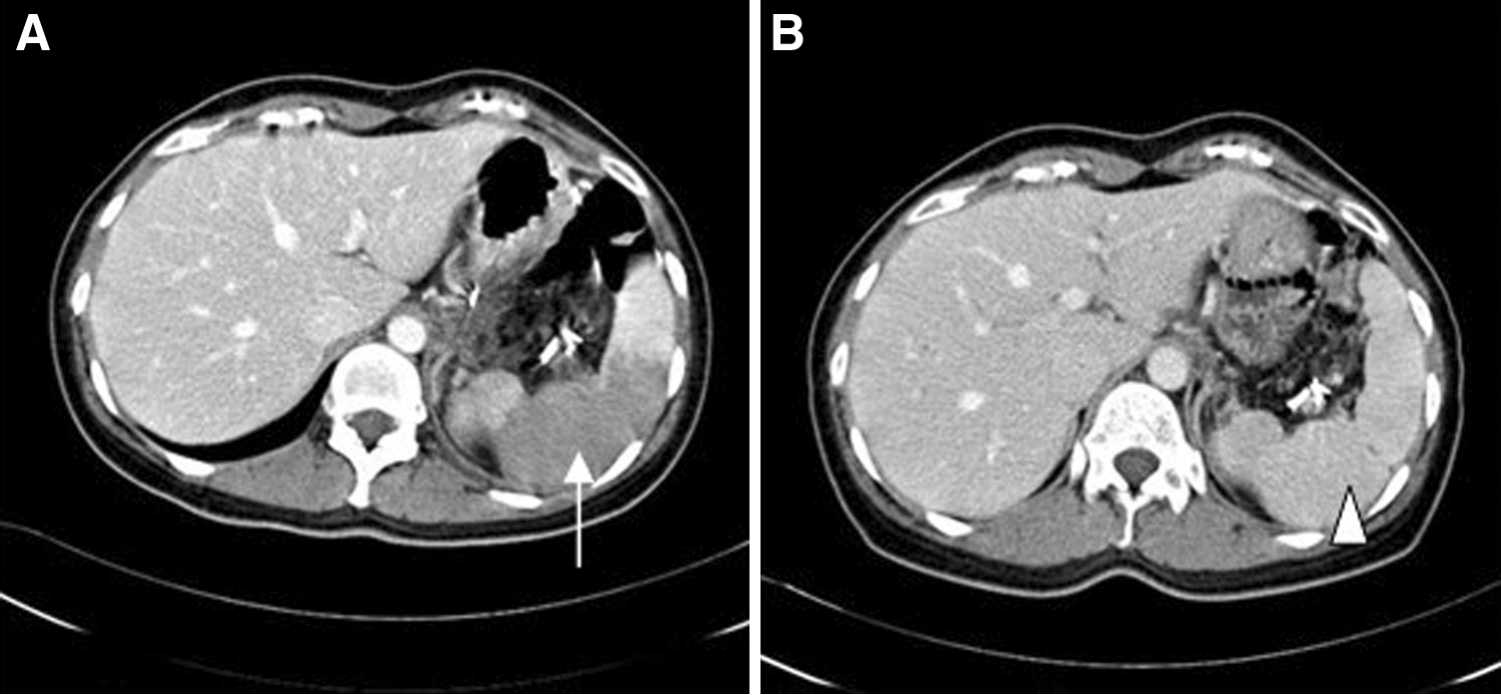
A patient with a grade 3 splenic infarction following lap-WT. A lap-WT was performed in this case to treat SPN at the body of the pancreas. A The CT scan showed a splenic infarction of greater than 50 % on postoperative day 3 (*white arrow*). B This recovered fully within 3 months, as indicated on follow-up CT scan (*white arrow head*)
